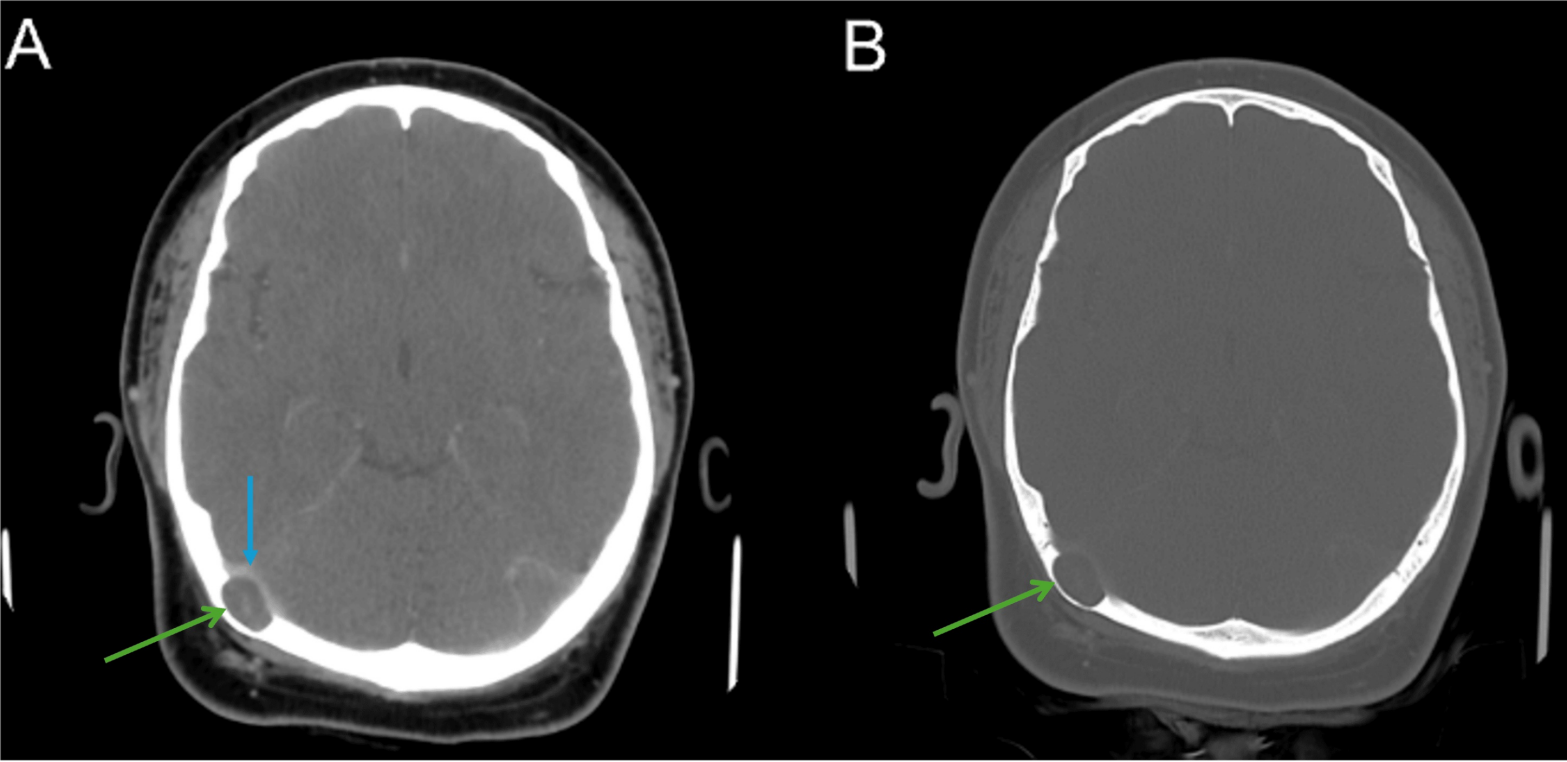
Soft-tissue (A) and bone (B) algorithm axial contrast-enhanced CT images of the head demonstrate a 1.8 cm intradiploic lucent lesion (green arrows) within the right occipital calvarium. The lesion markedly thins the inner table (B) and exerts mass effect on the right transverse sinus (blue arrow), suggesting extrinsic venous compression.
